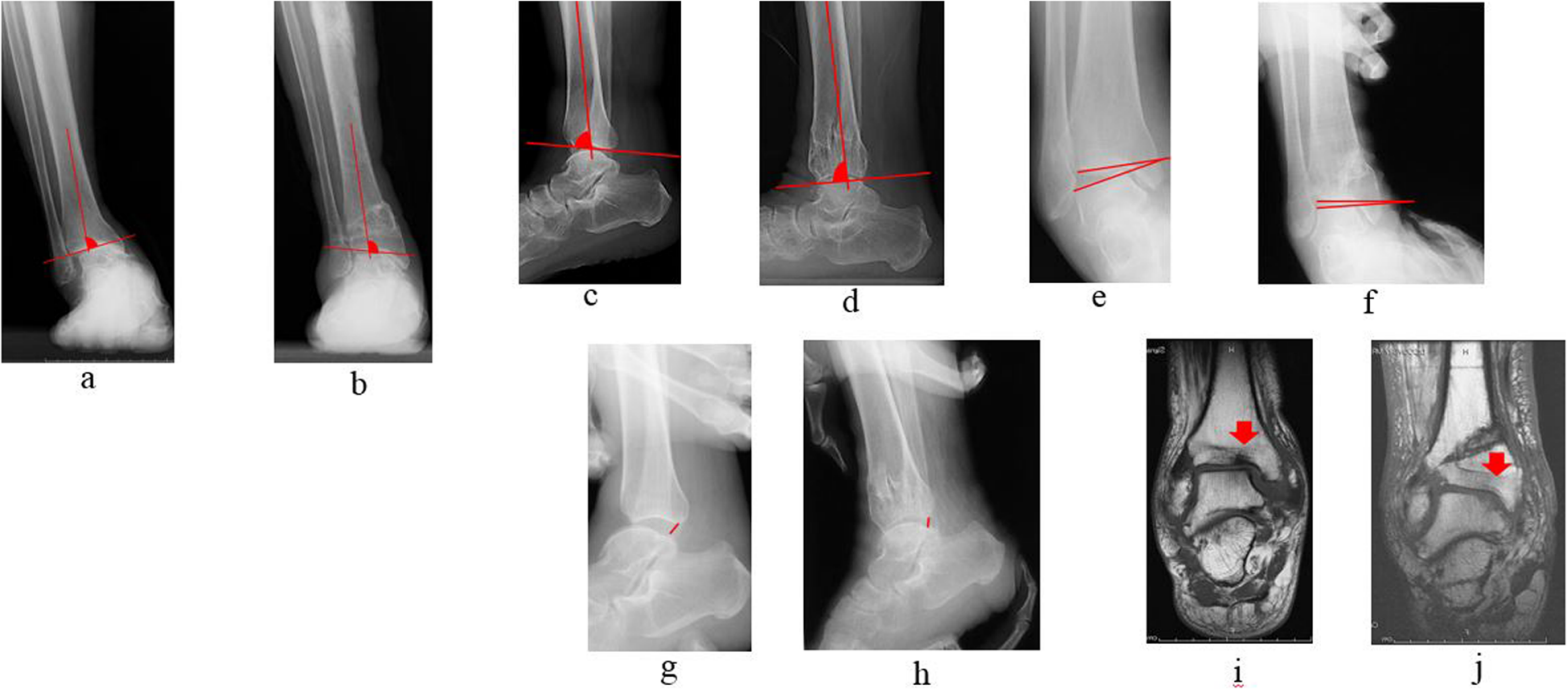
Pre- and postoperative images. a Preoperative antero-posterior mortise angle on X-ray. b Postoperative antero-posterior mortise angle on X-ray. c Preoperative lateral mortise angle on X-ray. d Postoperative lateral mortise angle on X-ray. e Preoperative talar tilt angle on ankle stress radiography. f Postoperative talar tilt angle on ankle stress radiography. g Postoperative anterior translation of the talus on ankle stress radiography. h Postoperative anterior translation of the talus on ankle stress radiography. i Signal changes (arrow) observed on magnetic resonance imaging (MRI) before osteotomy. j Signal changes (arrow) on MRI disappeared after osteotomy
